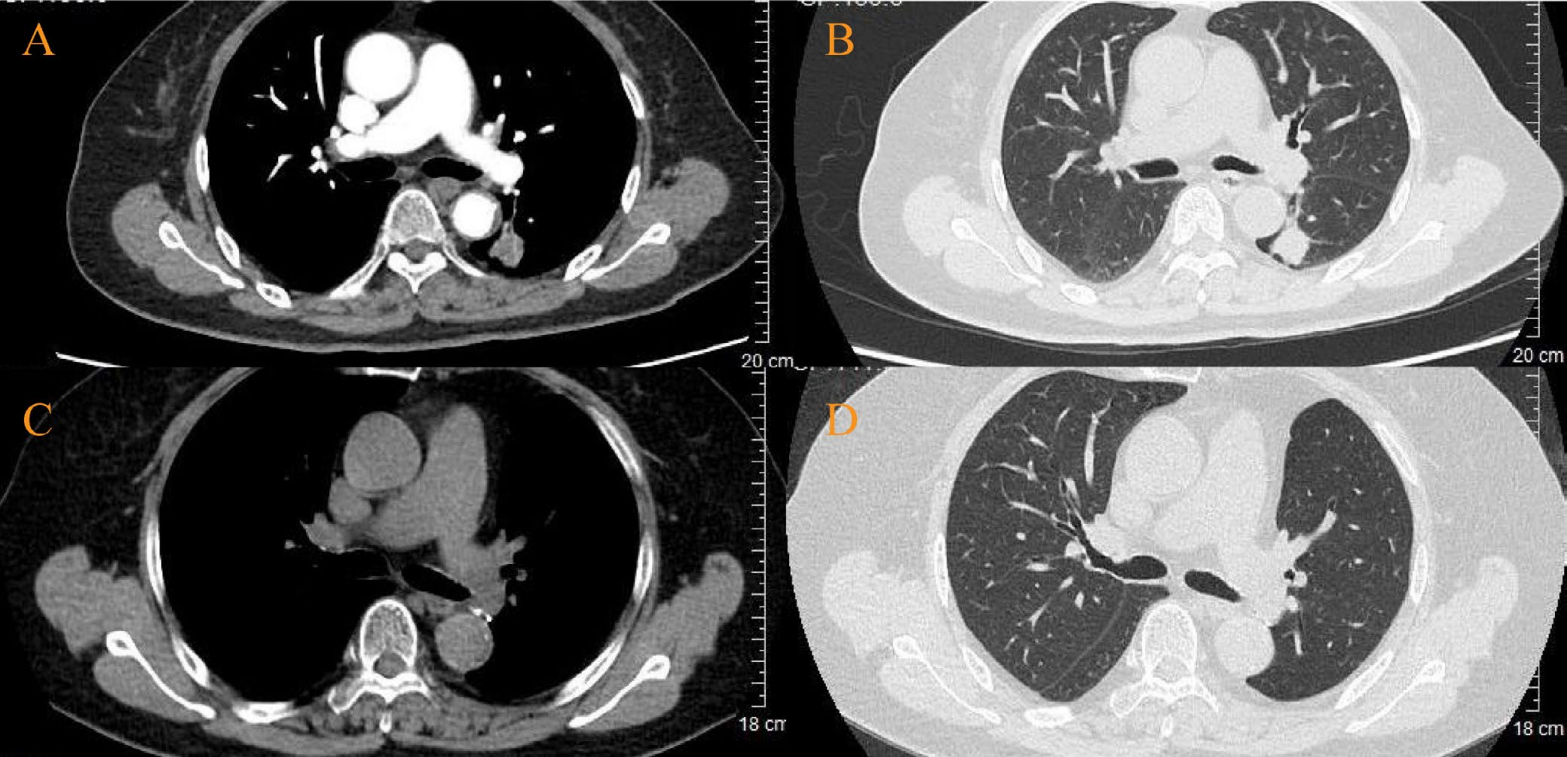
Radiologic evolution of left lower lobe lung tumor pre‐ and post‐surgical resection. (A, B) Baseline contrast‐enhanced axial chest CT at diagnosis. (C, D) Postoperative surveillance CT at 34‐month follow‐up.
